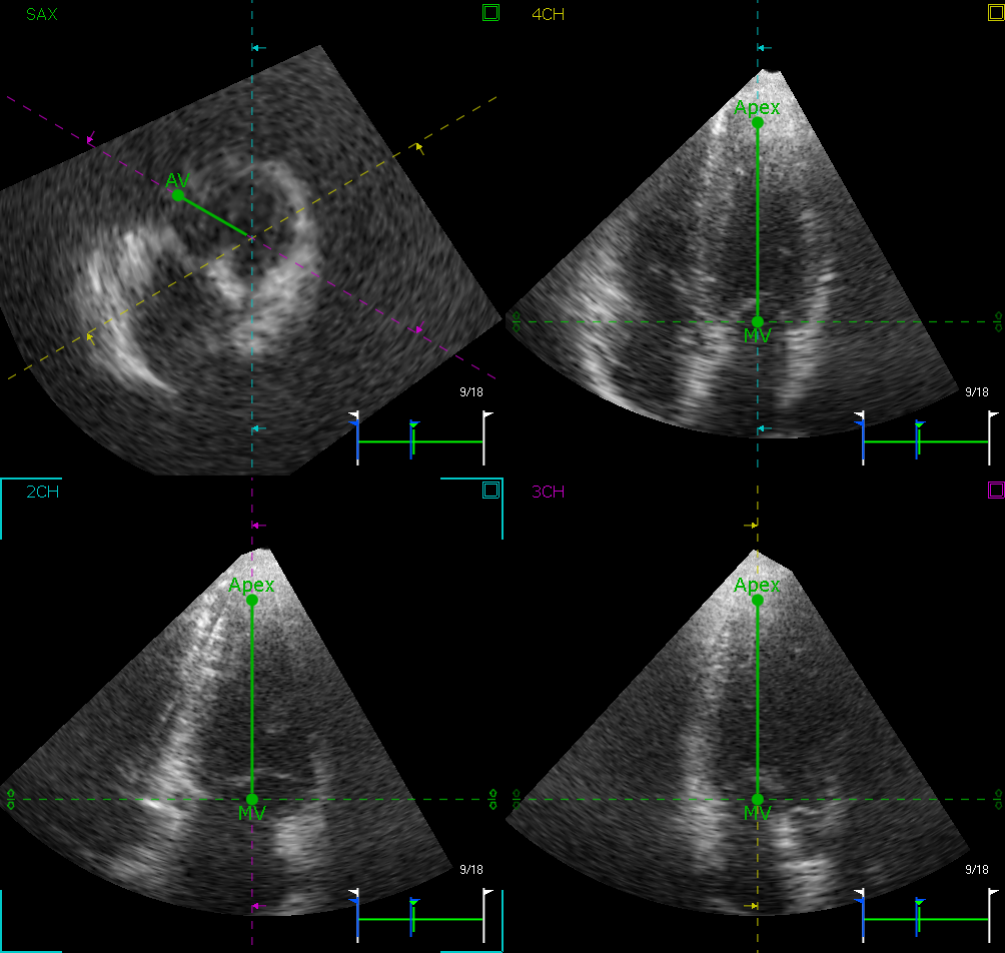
Three-dimensional speckle-tracking echocardiography (3D STE) offline analysis. First, the long axis (LAX) view is selected. The mitral valve landmark is placed at the annular level in the middle of the mitral valve, and the apical landmark is placed at the apex. Then, the short-axis view is selected. The aortic valve landmark is placed at valve level in the middle of the aortic valve. Manual tracking revision is performed if needed.
